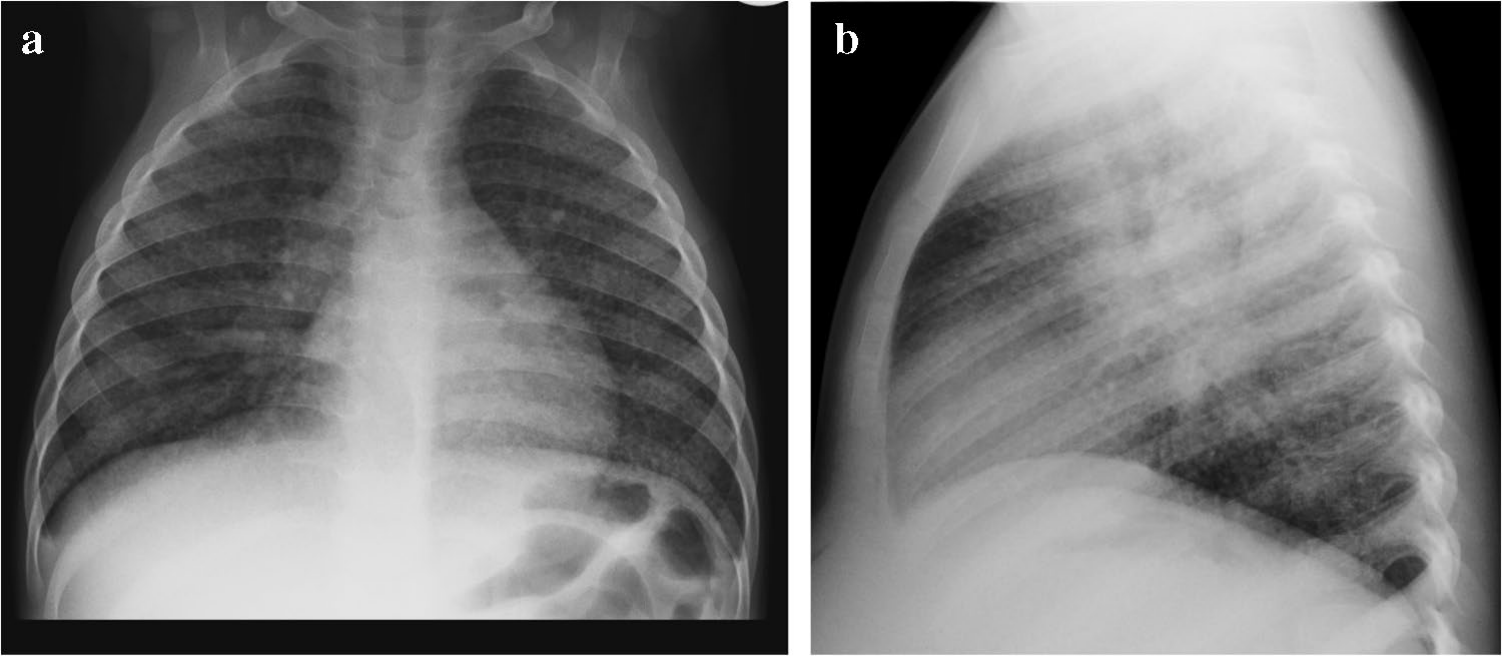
Posteroanterior (a) and lateral (b) chest radiographs in a 5-year-old boy demonstrate a diffuse pattern of miliary nodules in keeping with haematogenous spread of tuberculosis. Note the leftward displacement of the trachea and prominent right hilum, representing paratracheal and hilar lymphadenopathy
